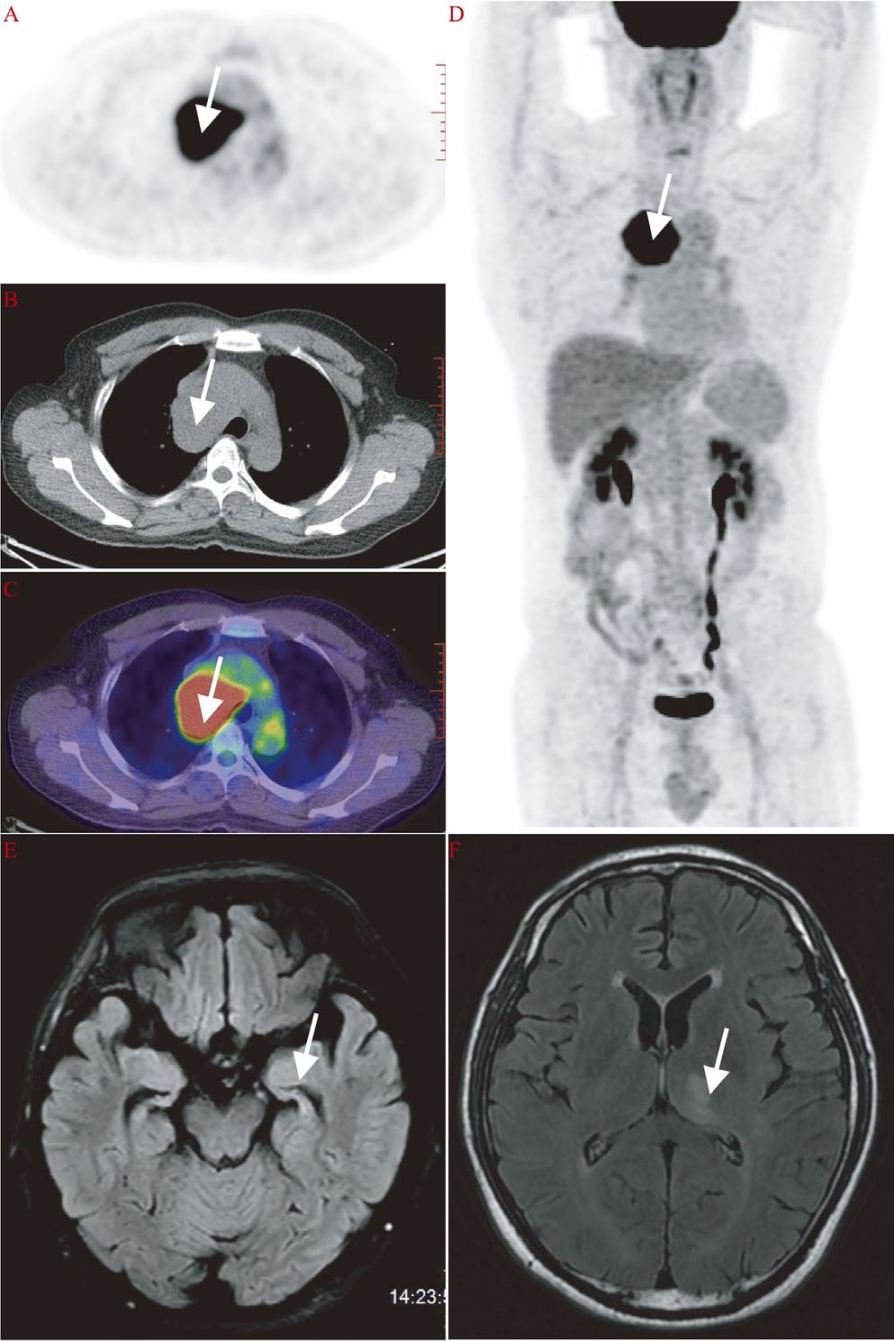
Representative MRI and ^18^F‐FDG PET/CT findings in patients with anti‐AMPAR encephalitis. Whole‐body ^18^F‐FDG PET/CT imaging of Patient 2 revealed a hypermetabolic pulmonary lesion consistent with lung cancer, indicated by white arrows (A–D). Brain MRI of Patient 3 demonstrated bilateral hyperintense signals in the medial temporal lobes on T2‐FLAIR sequences, predominantly affecting the hippocampi (E). In Patient 7, T2‐FLAIR imaging showed a focal hyperintensity in the left thalamus (F), suggestive of localized inflammation or metabolic dysfunction.
